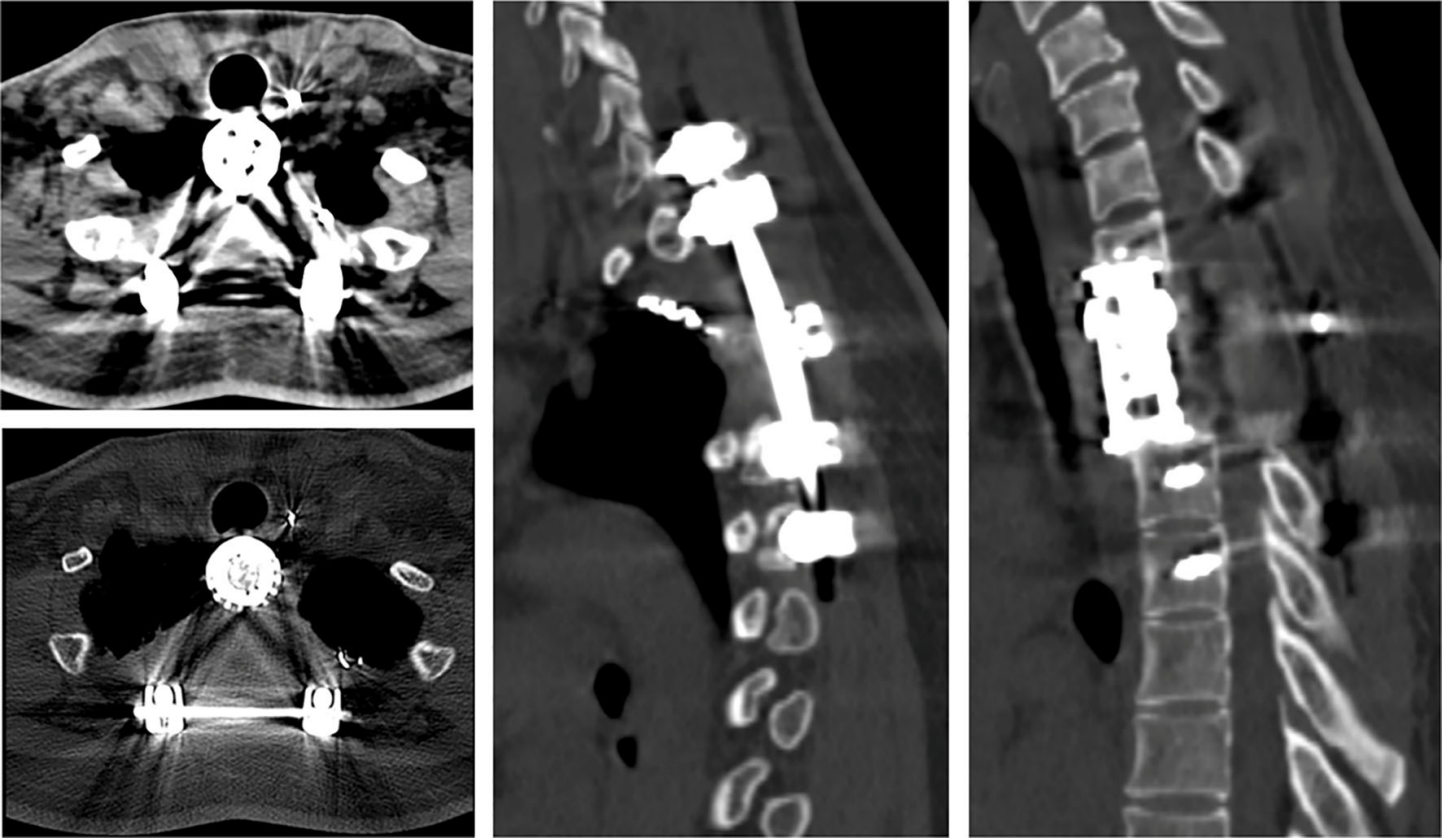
CT imaging reveals no evidence of recurrence throughout the 2-year follow-up period, with internal fixation in place.
